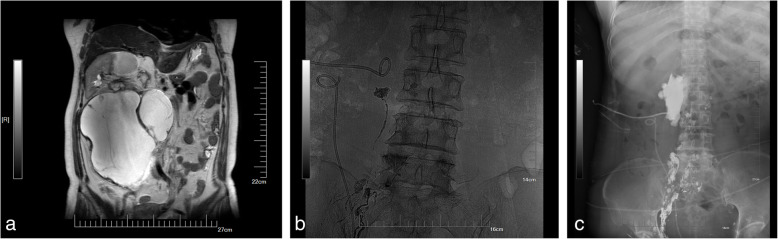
Intranodal lymphangiography and embolisation were performed to treat lymphatic leakage after abdominal surgery (patient 3). a Preoperative MRI shows a large cystadenoma with a maximum diameter of 16 to 18 cm. b The white arrow is a drainage tube placed at the time of surgery. The white star shows the leakage of iodized oil and aggregation on the right side of the spine. c The mixture was slowly injected at the site (black star) under fluoroscopy
